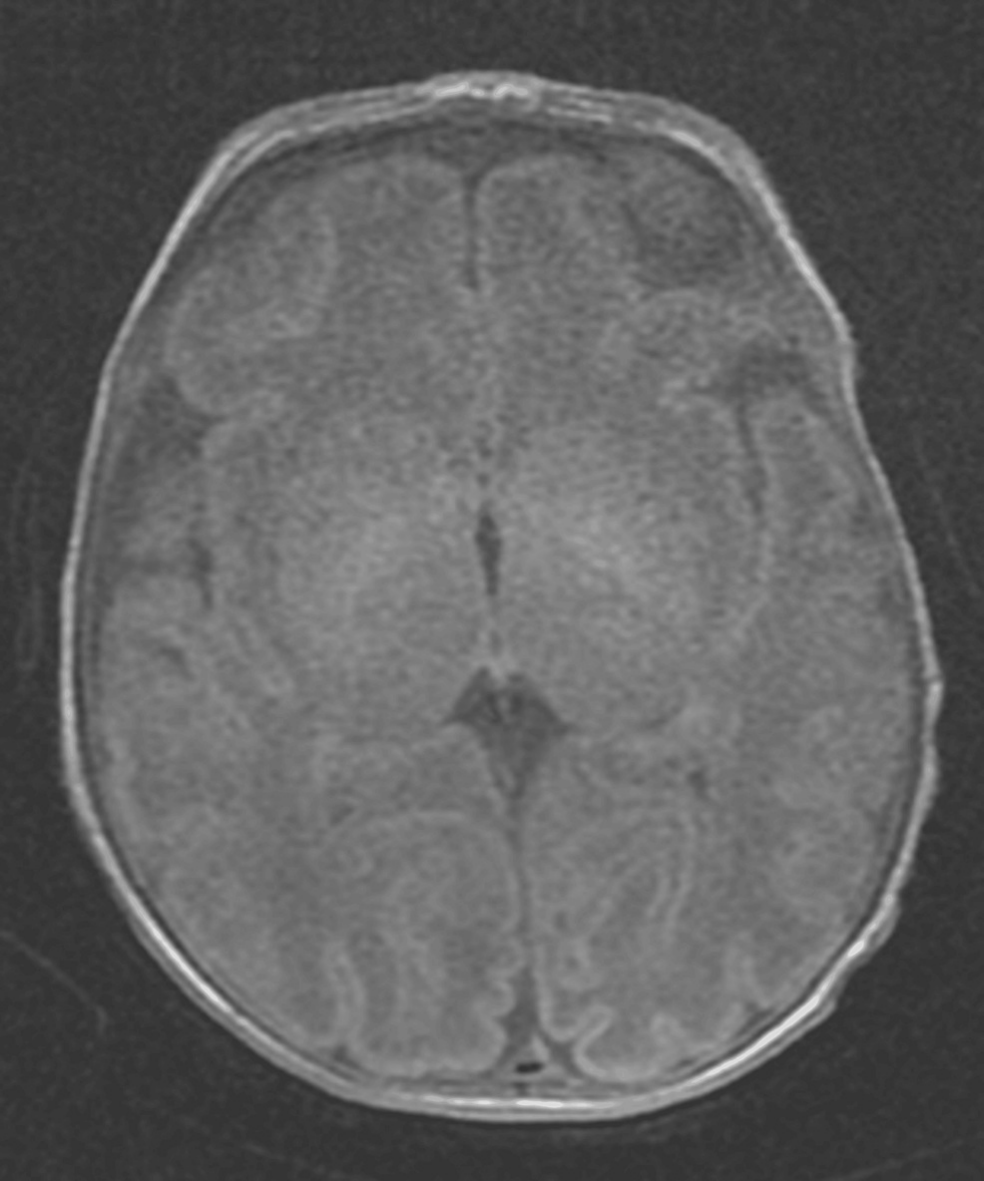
Normal brain MRI of our patient
